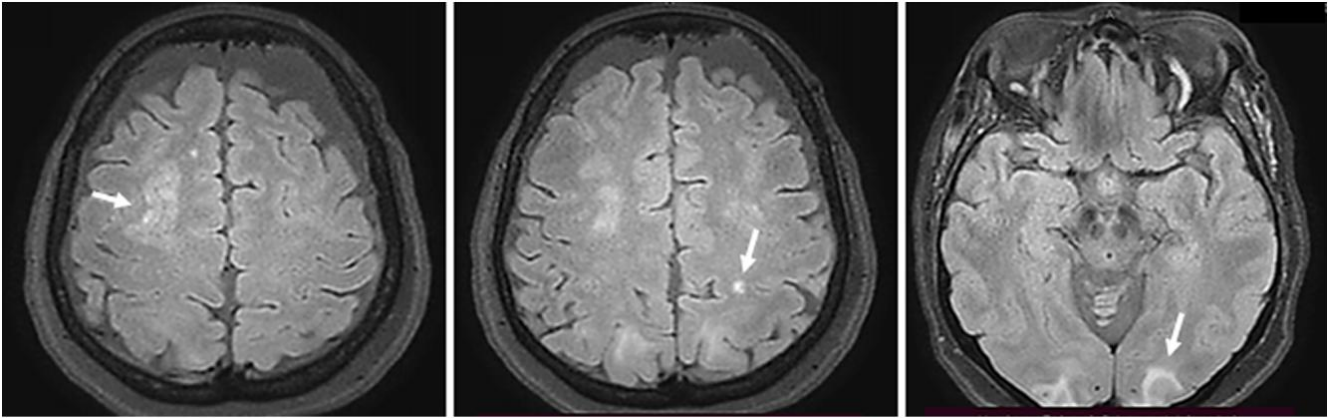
Brain MRI findings of the patient at the time of diagnosis, with bilateral cortical and subcortical hyperintense lesions (arrows) of both occipital, parietal, and right frontal lobes in T2-weighted or FLAIR sequences, diagnostic for PRES.
